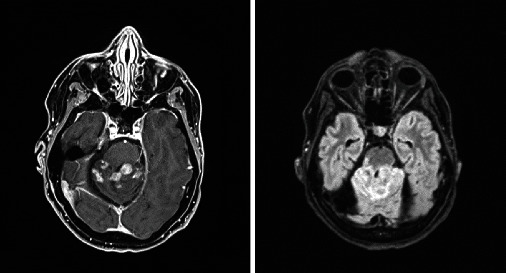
Multiple enhancing masses throughout the brain. Radiographic differential diagnosis: multiple metastatic lesions.
